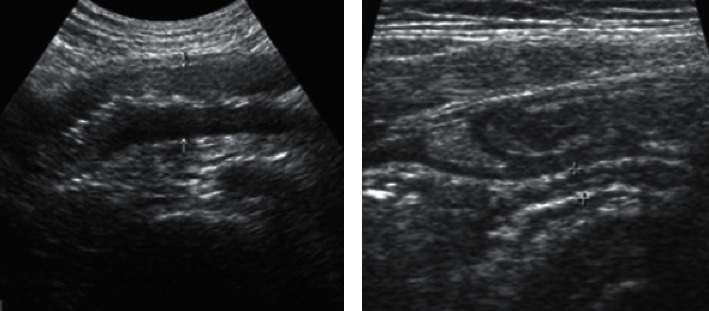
Intestinal ultrasound images of a child patient. (a) The image of a child, female, 10 years old, suffered from abdominal pain and diarrhea for 2 weeks. (b) The image of a child, male, 9 years old, suffered from a tight abdomen, obvious diarrhea, and abdominal pain.
